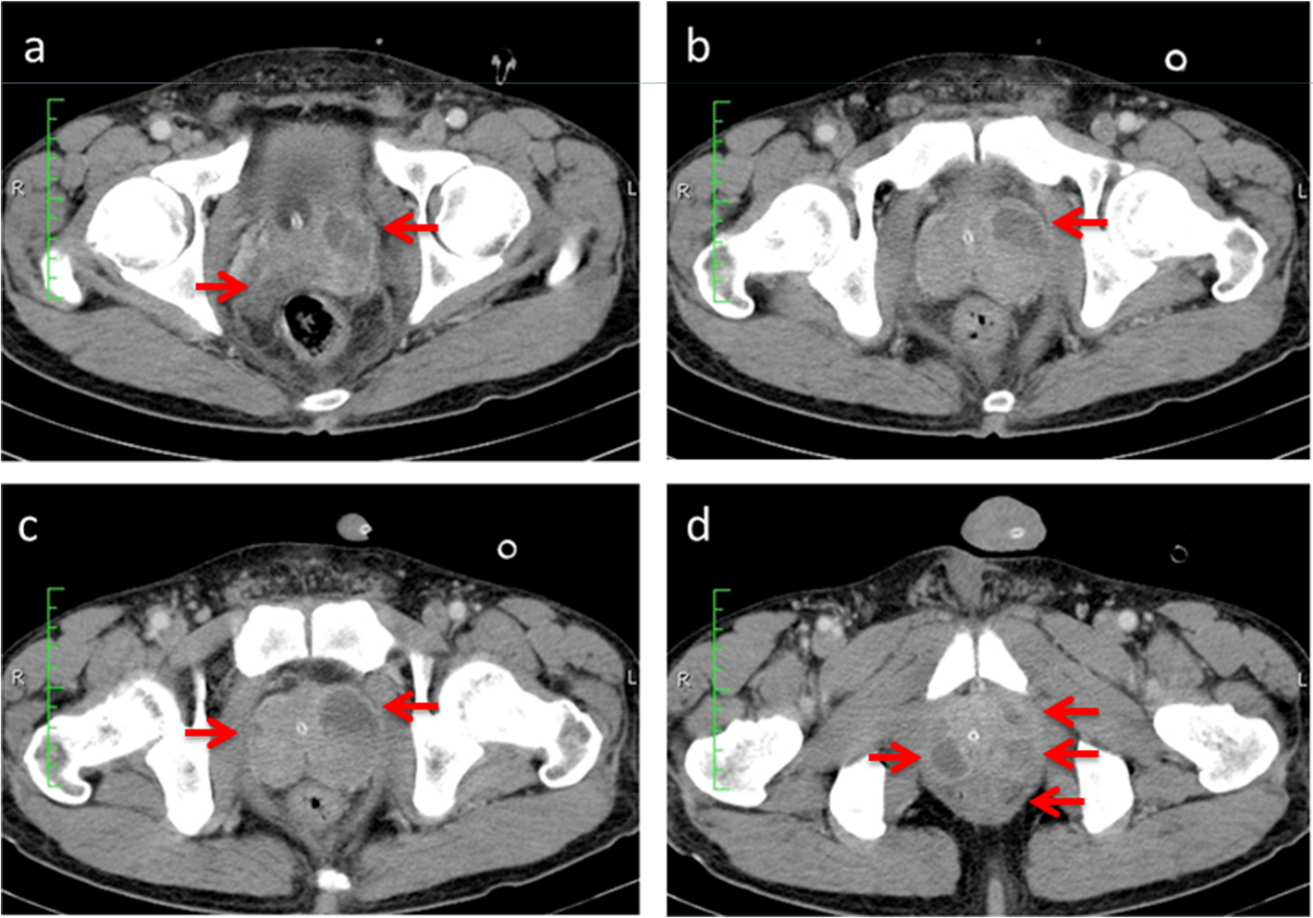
Contrast-enhanced computed tomography scan, revealing multiple large prostatic abscesses (red arrows). In sequence from (a) to (d), the images are sliced from the head side to the foot side
